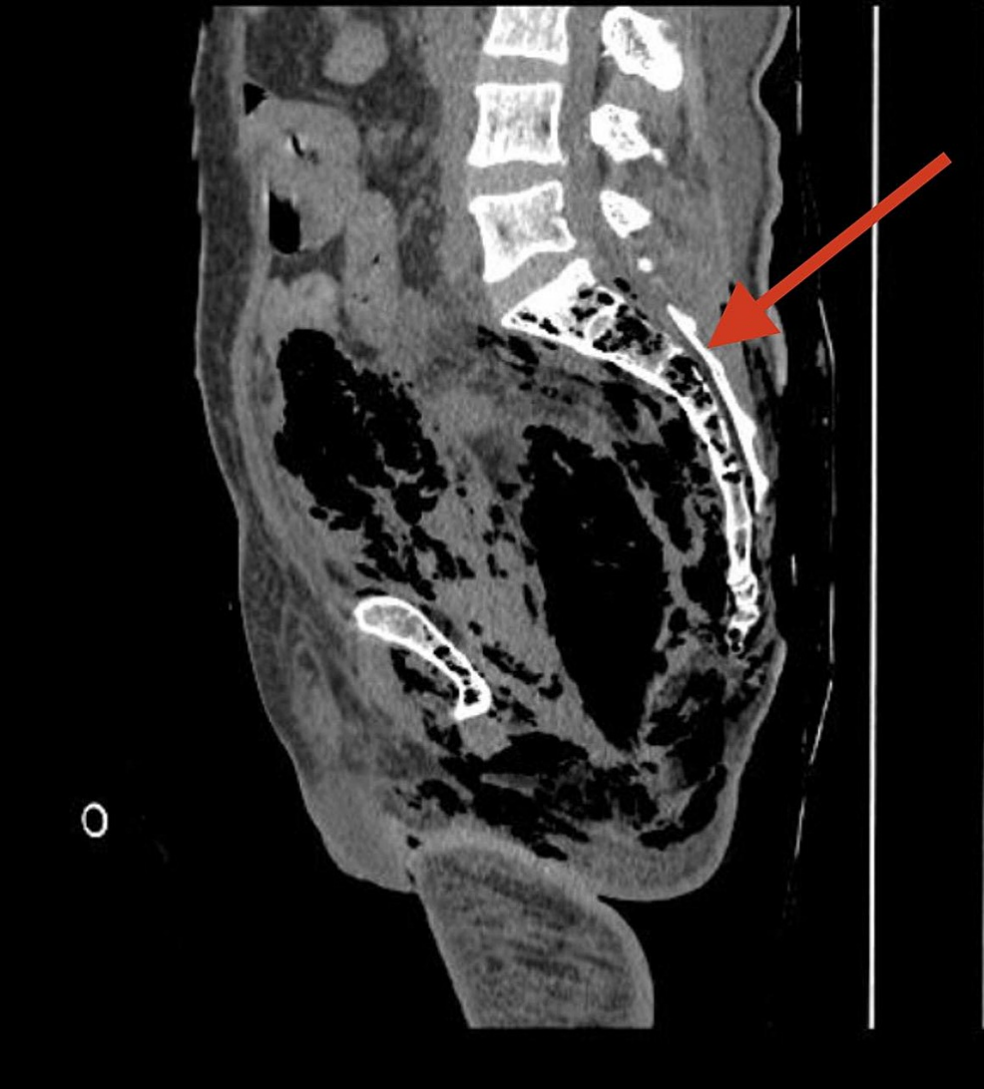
A contrast-enhanced CT of abdomen showing intramedullary air foci within the sacrum (red arrow).
